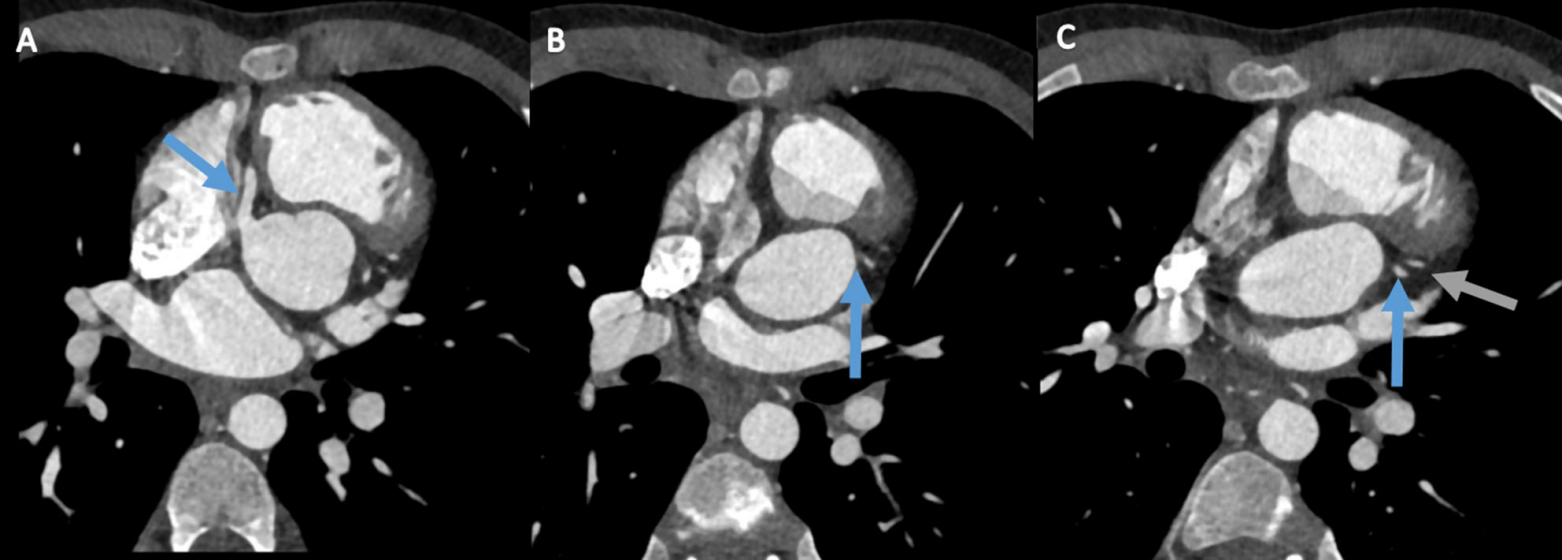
Cardiac CTA displaying a normal course and filling of the RCA (A, blue arrow). There is a stenotic origin of the LCA (B, blue arrow). More distal there is filling of the LAD (C, gray arrow) and Cx (C, blue arrow)
